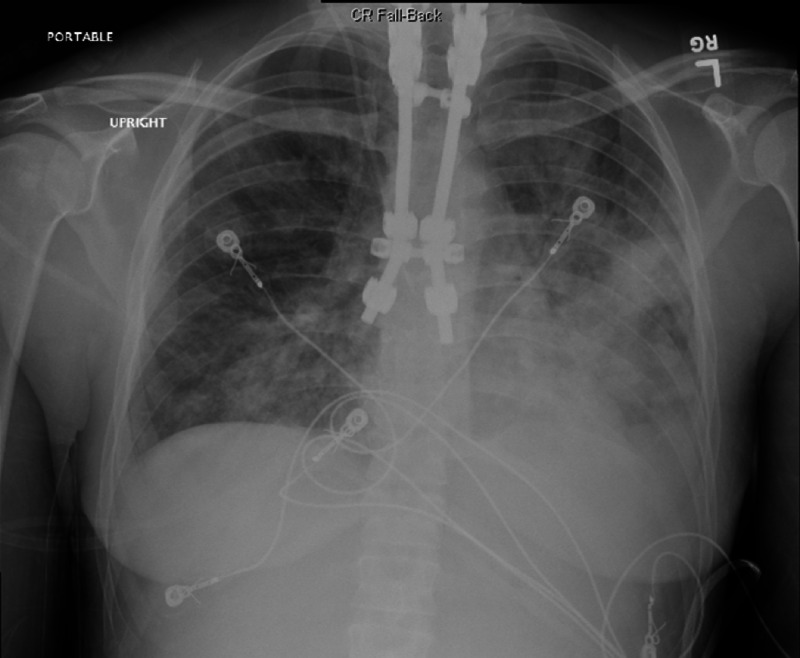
Chest radiograph on hospital day 5 showing interval decrease in bilateral airspace opacities, representing an improvement in bilateral pneumonia.
